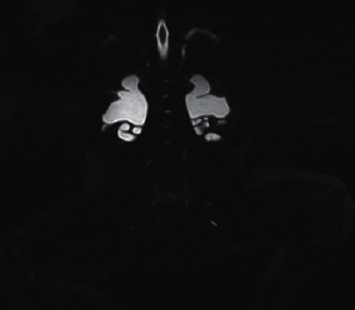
MRI showed bilateral pelvicalyceal dilation and tortuous ureters.
